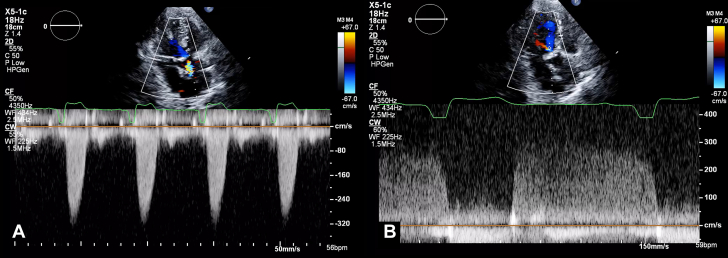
Apical 3-Chamber View Continuous -Wave Doppler Assessment Apical 3 chamber: align CW Doppler through the aortic valve to obtain aortic valve peak velocity, gradients, and Doppler assessment of aortic regurgitation.
